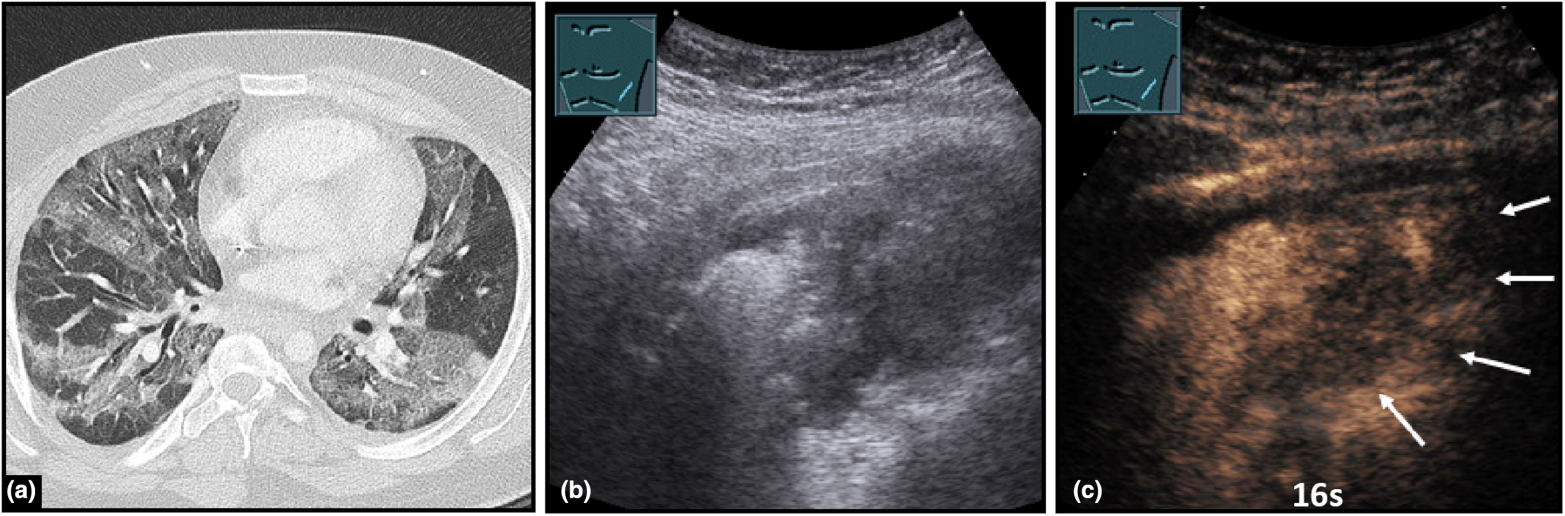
A 23‐year‐old patient presented with COVID‐19 pneumonia. Visualisation of bilateral, milky‐glass‐like pulmonary infiltrates on computed tomography (courtesy of Prof. Dr. Andreas H. Mahnken, Department of Radiology, University Hospital Marburg) (a); B‐mode ultrasound (b) shows a hypoechoic, irregularly bordered consolidation; contrast‐enhanced ultrasound (c) reveals an inhomogeneous perfusion with areas of reduced/absent perfusion (arrows) after 16 seconds, indicating the presence of COVID‐19 pneumonia with impaired perfusion.
